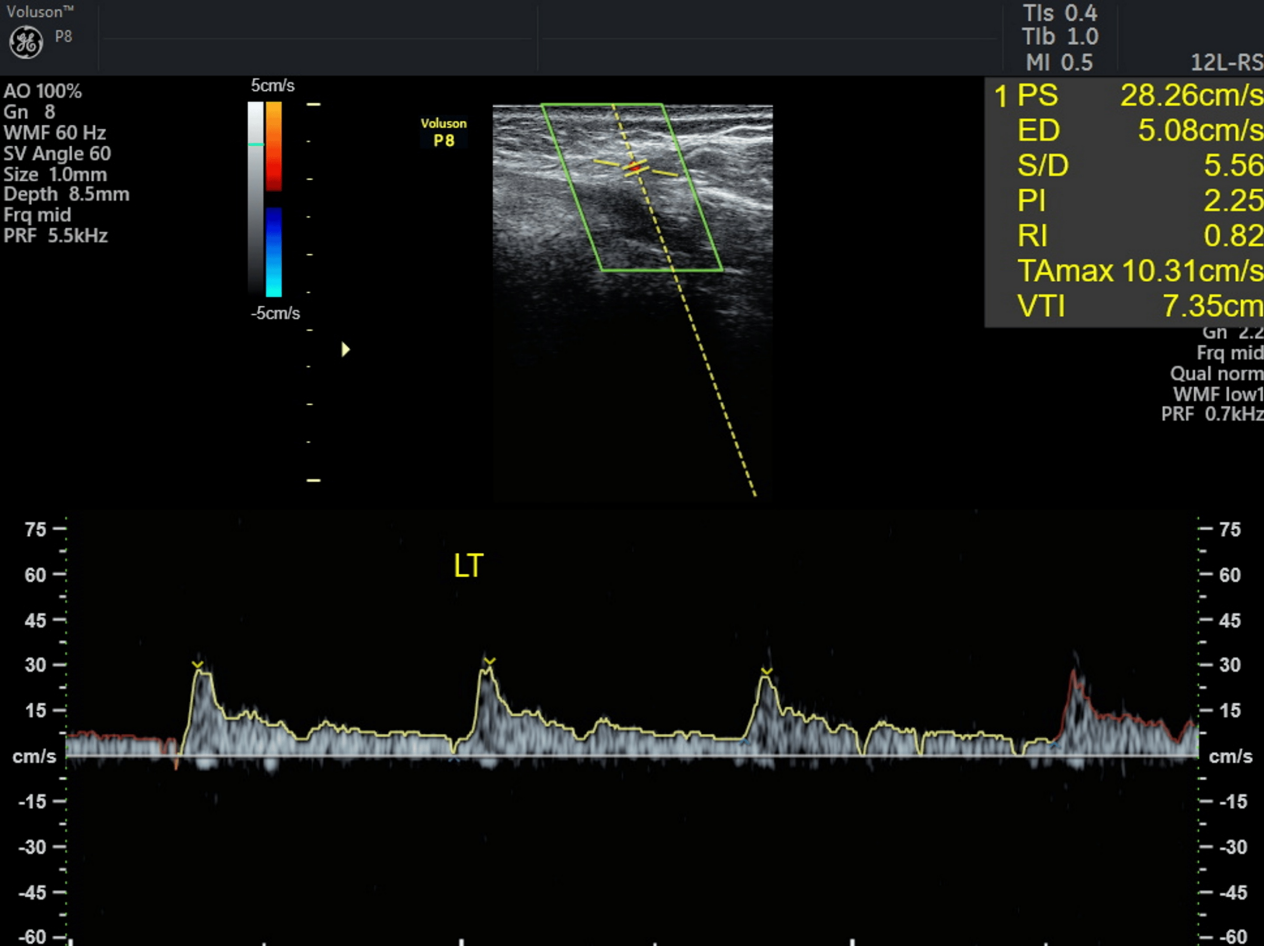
Ultrasound Doppler flowmetry of CAF + CTG on the fifth day. CAF: coronally advanced flap, CTG: connective tissue graft, PS: peak systolic velocity, ED: end-diastolic velocity, PI: pulsatility index, RI: resistive index, VTI: velocity time integral, LT: left.
